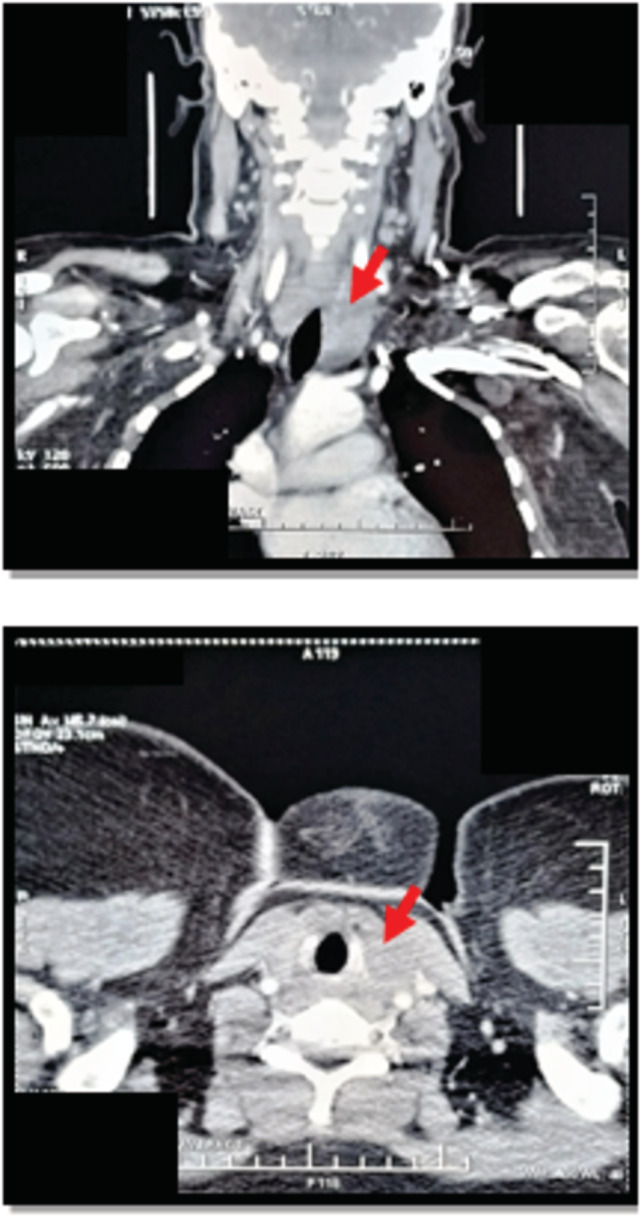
Cervico‐thoracic computed tomography scan showing the left thyroid lobe plunging into the mediastinum.
